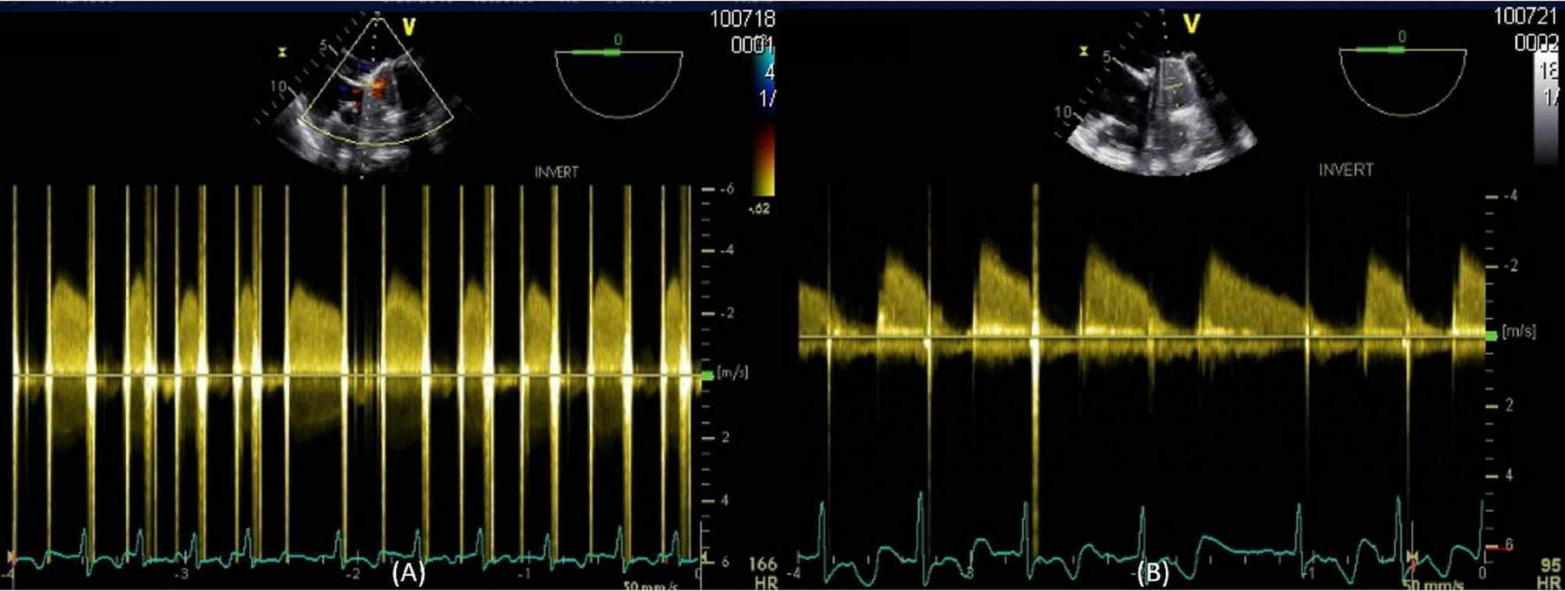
Trans-esophageal echocardiogram with continuous wave doppler across the mitral valve. Demonstrating elevated mean trans-mitral gradient of 24 mmHg (averaged over five beats) at heart rate of 166 beats/min before initiation of thrombolytics (A) and a trans-mitral gradient of 15 mmHg (averaged over five beats) at a heart rate of 95 beats/minute after trial of thrombolytics (B).
